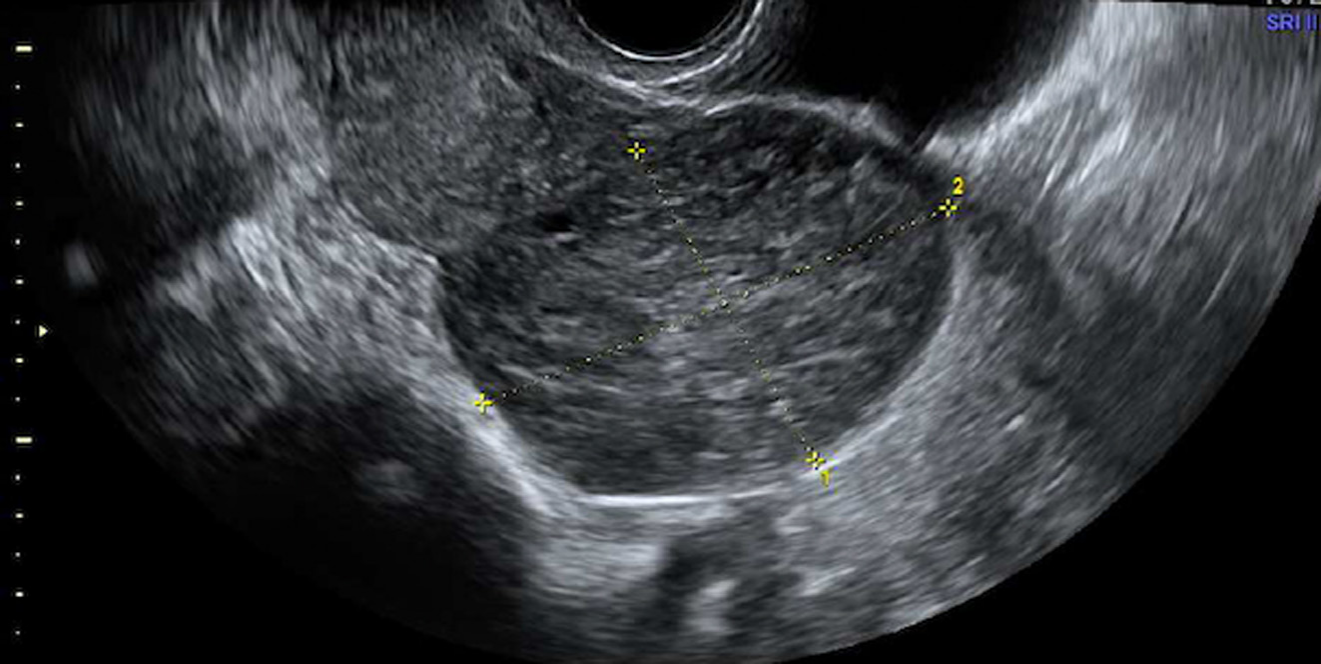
Maximum diameter at first diagnosis at ultrasound of case 1.
